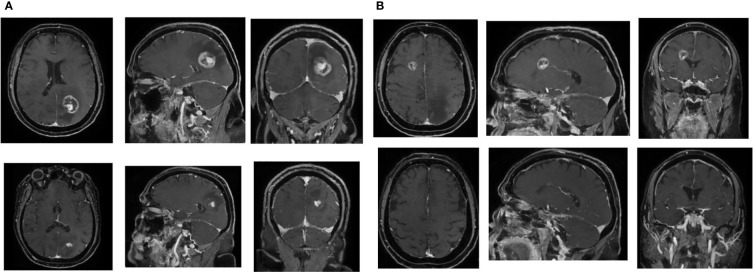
Impressive volume reduction of two brain radionecrosis areas. (A) Left occipital lesion. Above, MRI before cabozantinib (9 months after SRS). Below, MRI after cabozantinib (13 months after SRS). (B) Right frontal lesion: Above, MRI before cabozantinib (9 months after SRS). Below, MRI after cabozantinib (13 months after SRS).
